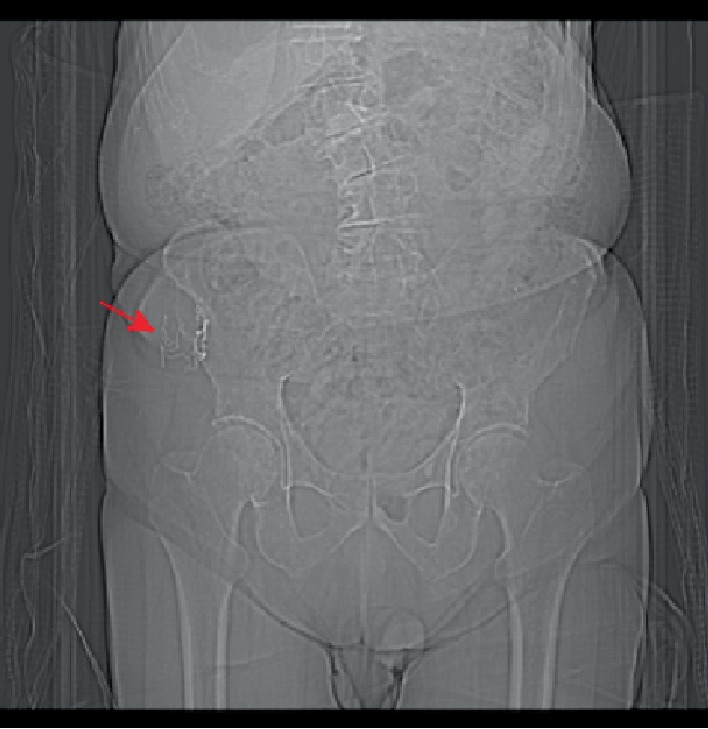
CT scan (scout image) demonstrating radiopaque strings in the patient's right hip.
